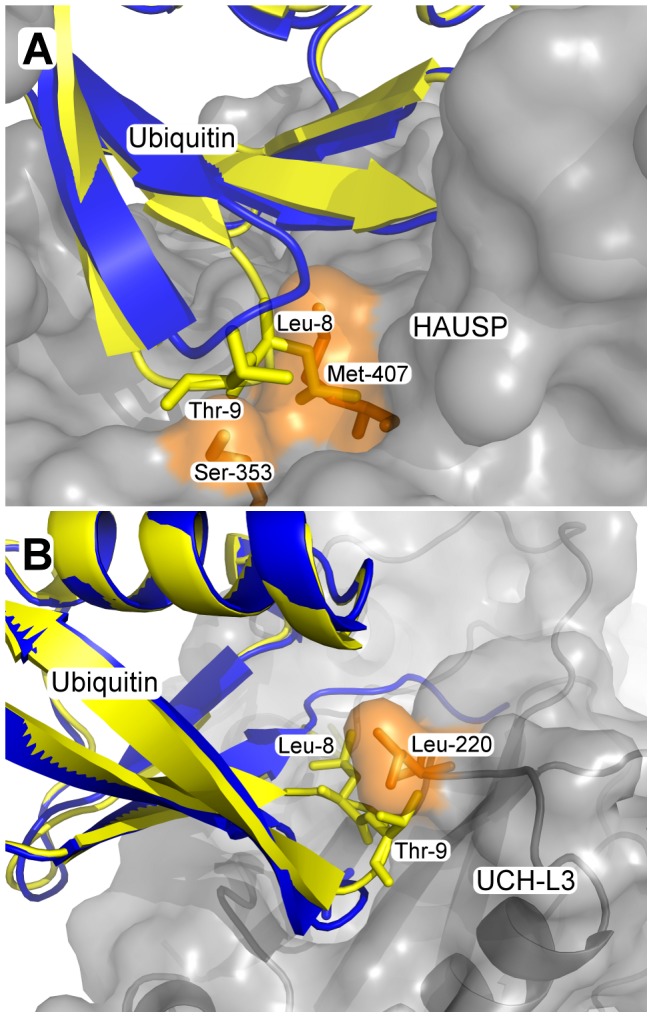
Steric clashes restricting pincer mode dynamics in the complex. Detail from the xray structure (A) 1nbf (ubiquitin bound to HAUSP) and (B) 1xd3 (ubiquitin bound to UCH-L3). For each structure, the compatible ubiquitin structure is shown in blue, while an incompatible structure that has been fitted to the same position is shown in yellow. Clashes with the binding partner are marked in orange.
